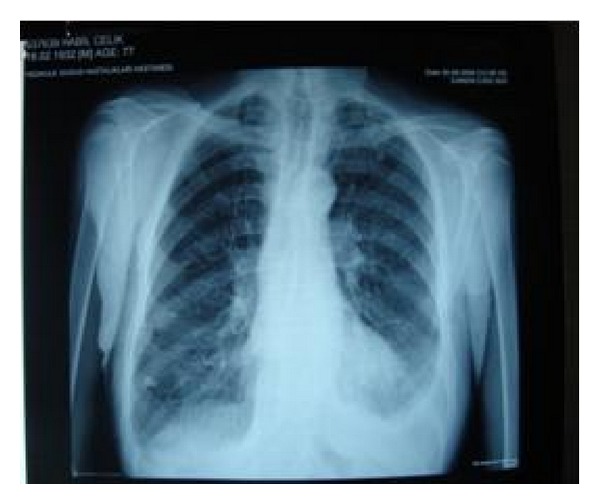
Chest radiography after treatment.
